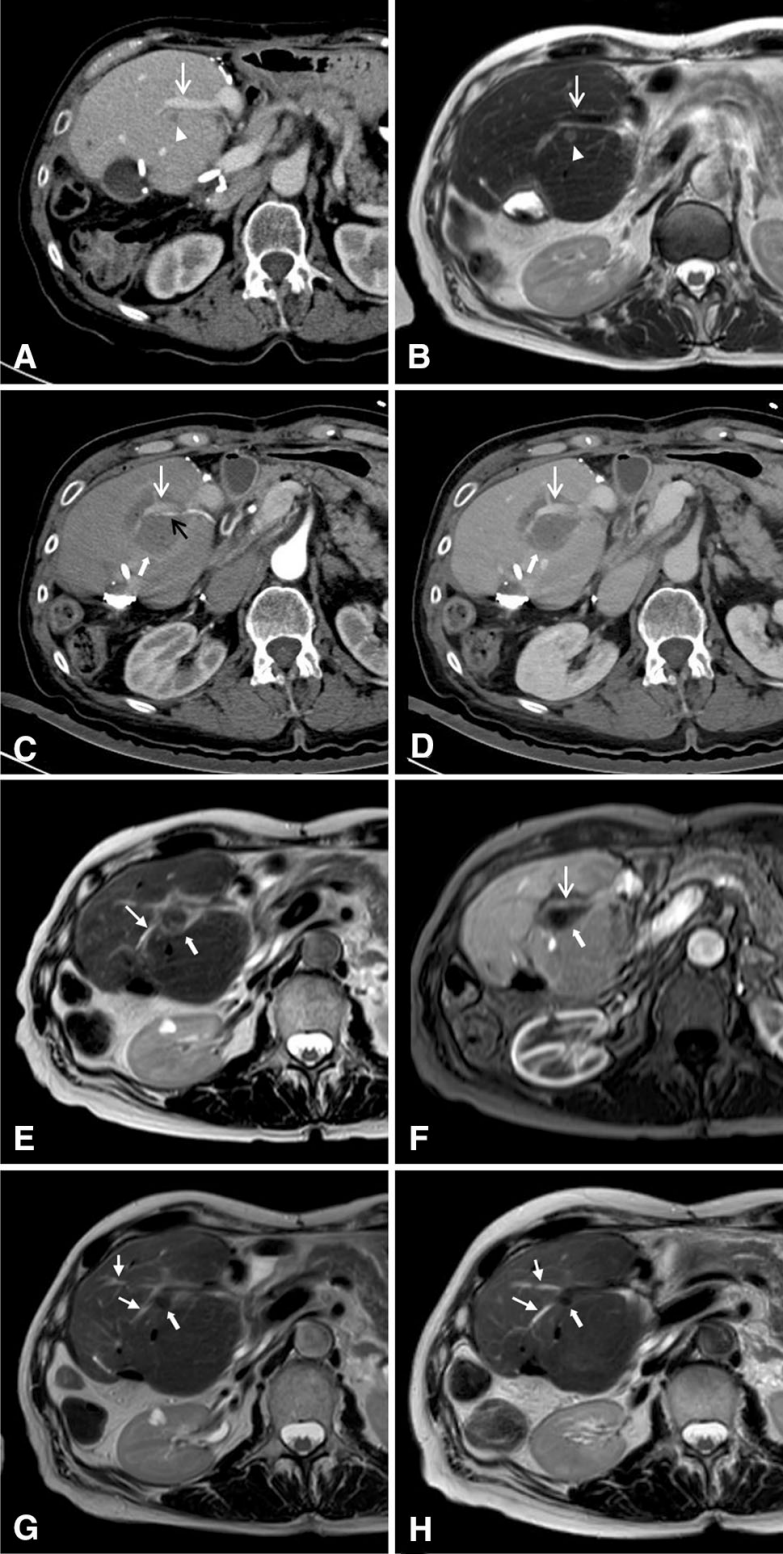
Sample case. 71-year-old female patient with synchronous metastatic colorectal cancer to the liver who priory underwent right hepatectomy and bisegmentectomy of segments II and III. 5 months after surgery the patient was diagnosed with a new metastasis in the liver remnant, which, accordingly, consisted of segments I and IV, only. A multidisciplinary tumor board made the decision to perform IRE. A (CT in venous phase) and B (MRI: T2w TSE) show the metastases (arrowhead) located immediately adjacent to the sole remaining portal vein branch of segment IV (white open arrow). C (CT in arterial phase) and D (CT in portal venous phase) show the ablation area immediately after the IRE procedure. The ablation zone (thick arrow) extends beyond the portal vein branch (white open arrow). The adjacent vessels, portal vein branch (white open arrow) and segment-IV-artery (black open arrow) remained perfused. E (MRI: T2w TSE) and F (MRI: T1w GRE in portal venous phase) show the ablation area 4 weeks after IRE. The ablation zone (thick arrow) has decreased in size. However, the patient now shows evidence of a subsegmental cholestasis (E, white closed arrow) due to an IRE-induced bile duct stricture. Plasma bilirubin remained within normal limits (0.6 mg/dl). The adjacent portal vein branch (white open arrow) is still perfused without evidence of vessel thrombosis. G and H (MRI: T2w TSE) show the ablation area 3 (G) and 6 (H) months after IRE. The subsegmental cholestasis (closed arrow) remained stable and did not require treatment (plasma bilirubin 6 months after IRE was 0.42 mg/dl). The ablation zone (thick arrow) further decreased in size. Follow-up imaging did not show any evidence of distant tumor recurrence in and outside the liver. IRE = Irreversible electroporation; CT = computed tomography; MRI = magnetic resonance imaging; T2w = T2-weighted; TSE = turbo spin echo; T1w = T1-weighted; GRE = gradient echo
